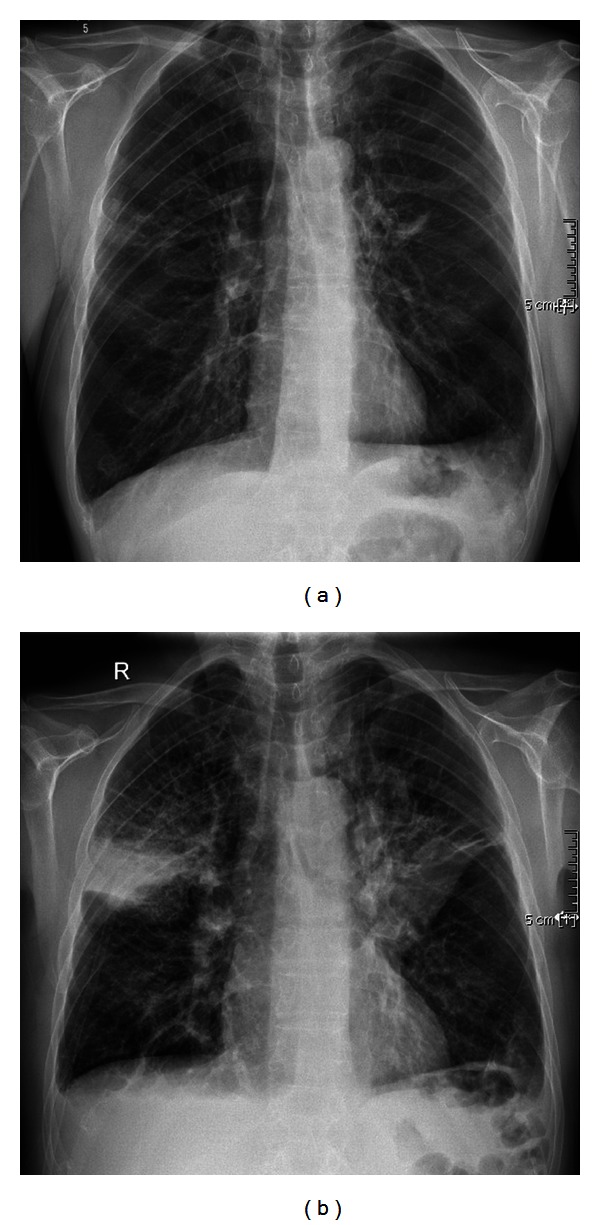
(a) Chest X-ray 4 weeks before the endoscopic lung volume reduction. (b) Chest X-ray 3 days after the endoscopic lung volume reduction shows atelectasis of both right and left upper lobe after endoscopic lung volume reduction.
